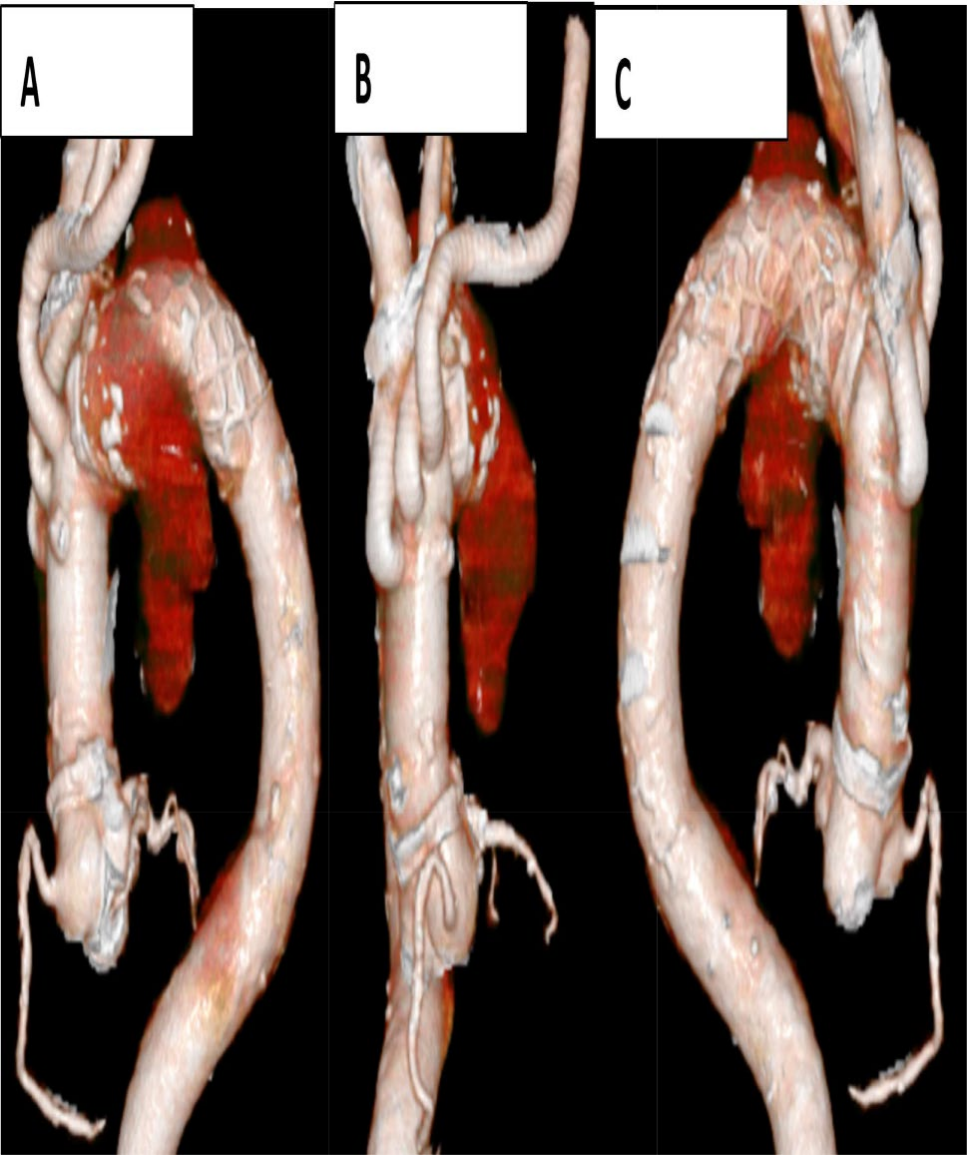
Postoperative 3D computed tomography
